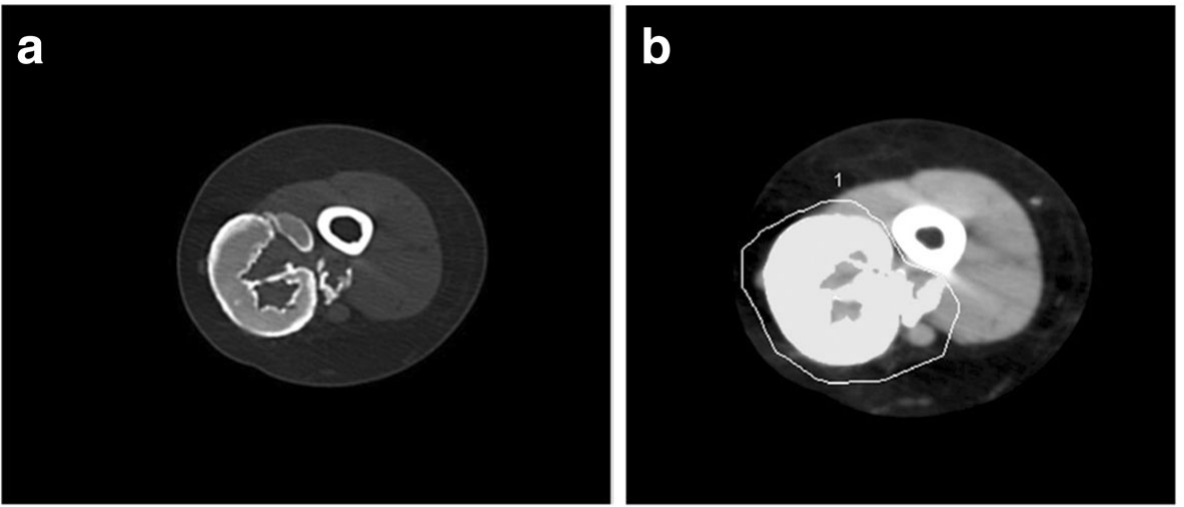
a Axial CT image of the upper arm of a child with JDM, showing a large cluster of calcifications medially. b The same axial CT image showing the calculated calcification volume highlighted in gray
